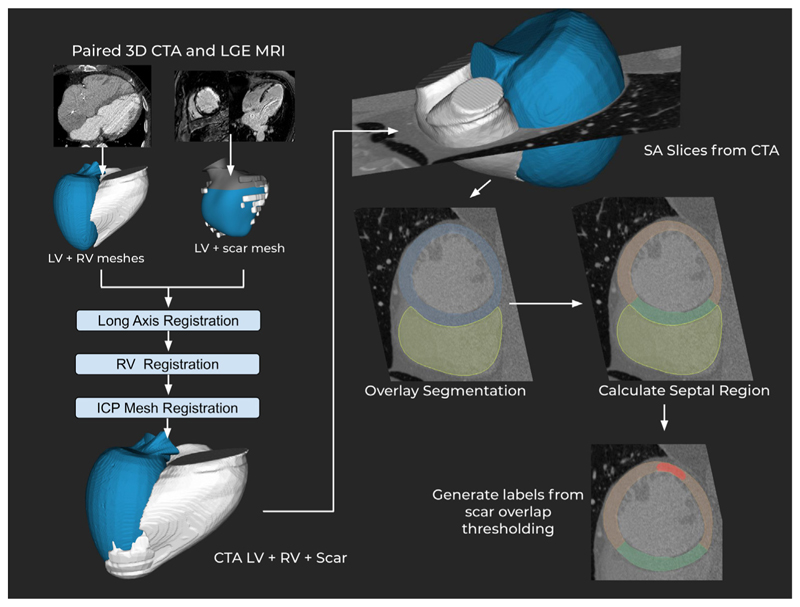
Data generation process for the septal/lateral scar CTA dataset. MRI meshes are registered to the CTA meshes in three steps. The meshes are aligned with the CTA DICOM volume, from which SA slices are taken. The septal area is calculated and scar class is determined by a threshold of volume against the volume of the region. ICP: iterative closest point registration.
